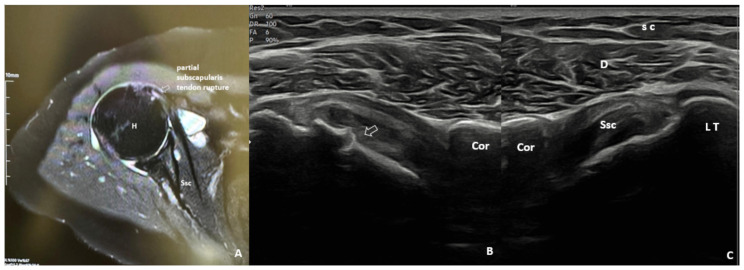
Axial MRI image demonstrating a partial rupture of the subscapularis tendon (A) Axial US image over the anteromedial shoulder reveals a partial subscapularis tendon rupture possibly due to the underlying cortical irregularities (arrow) of the lesser tuberosity (B) US image of normal contralateral side (C). Cor: Coracoid process; D: Deltoid; H: Humerus; LT: Lesser tuberosity; sc: Subcutaneous tissue; Ssc: Subscapularis tendon.
